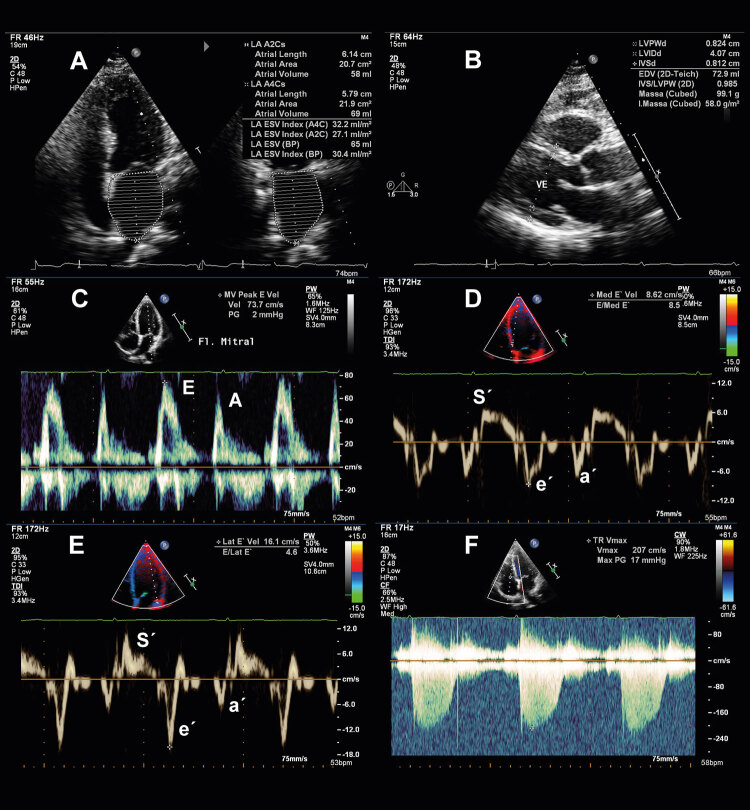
Critérios ecocardiográficos morfológicos (A e B) e funcionais (C a F) para aplicação do algoritmo diagnóstico para pacientes com suspeita de ICFEp. Os critérios morfológicos incluem a medida do volume indexado do átrio esquerdo (A), e o cálculo do índice de massa miocárdica e a espessura relativa de parede (B). Os critérios funcionais incluem a relação E/e’, calculada a partir da medida de onda E ao Doppler mitral (C) (v = 73,7 cm/s) e ondas e’ ao Doppler tecidual septal (D) (v = 8,6 cm/s) e lateral (E) (v = 16,1 cm/s), além da velocidade de pico do jato de regurgitação tricúspide (v = 2,07 cm/s) para estimar a medida da pressão sistólica de artéria pulmonar (F). v: velocidade.
